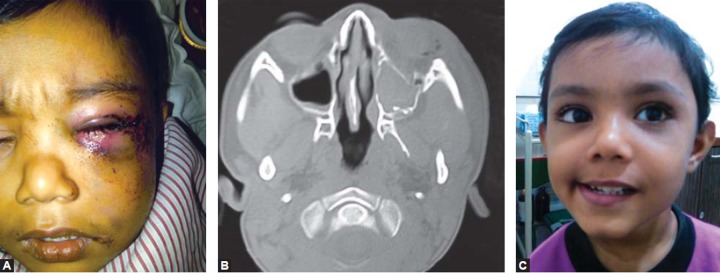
(A) History of fall of 3-year-old, (B) CT scan reveals left anterior wall of maxilla fracture, (C) 5 weeks postoperative
